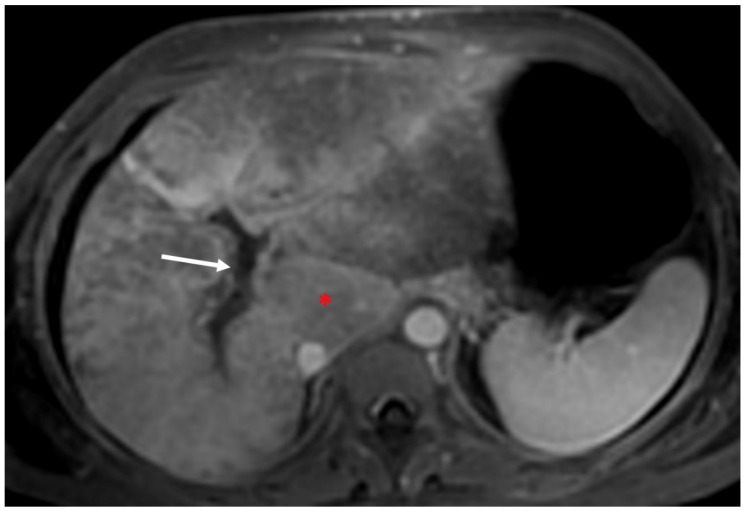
Axial portal venous phase MR image shows the coexistence of portal vein thrombosis (arrow) in patient with BCS. Notice the presence of enlarged caudate lobe (red asterisk).
